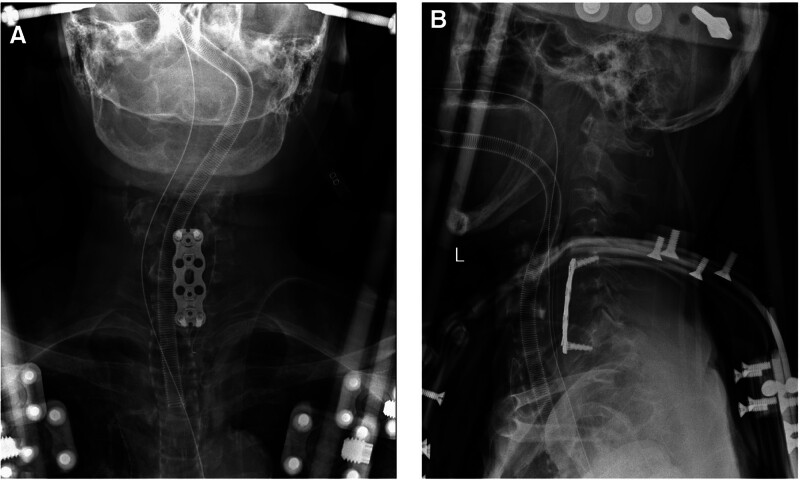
Cervical spine X-ray postanterior cervical corpectomy and fusion (ACCF). (A) the anteroposterior view; (B) the lateral view.
